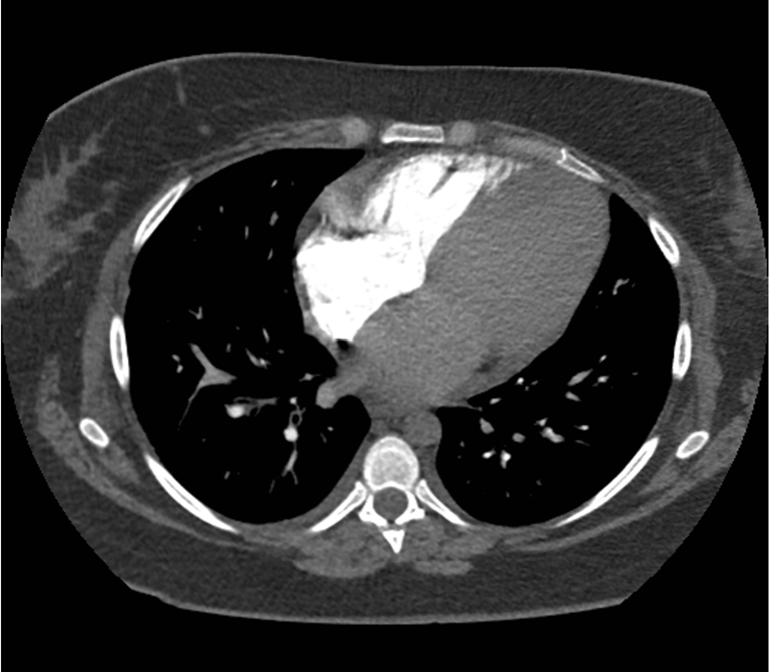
Cardiomyopathy resulting in poor contrast opacification of the left atrium, left ventricle and aorta on a thoracic CTA being obtained to evaluate for pulmonary embolism on a 64 slice CT. CECT demonstrates excellent opacification of the right heart, but poor opacification in the left heart related to prolonged pulmonary circulation time in a patient with left ventricular systolic dysfunction. BNP was immediately obtained and was elevated at 23,000, echocardiogram obtained within the next 4 h demonstrated a left ventricle EF of 22 %
